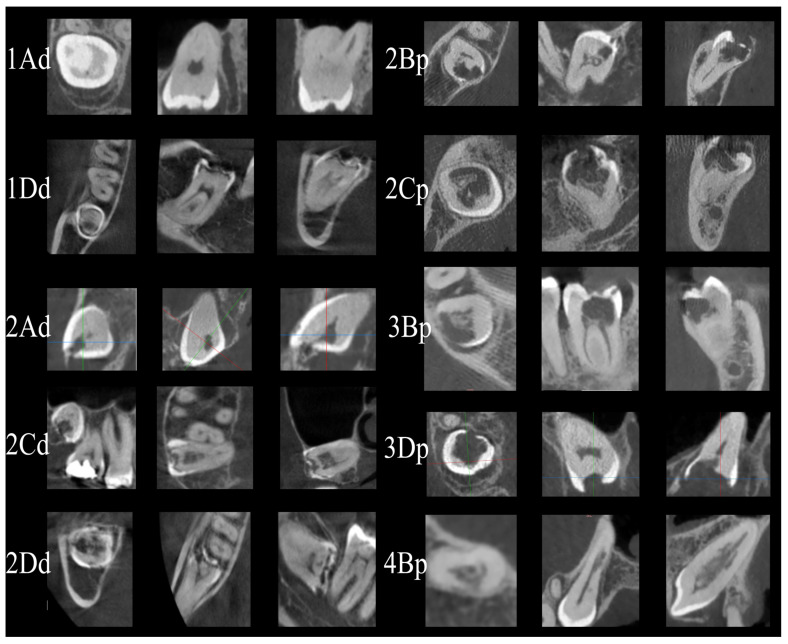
CBCT images of various types of PEIR according to novel classification.
